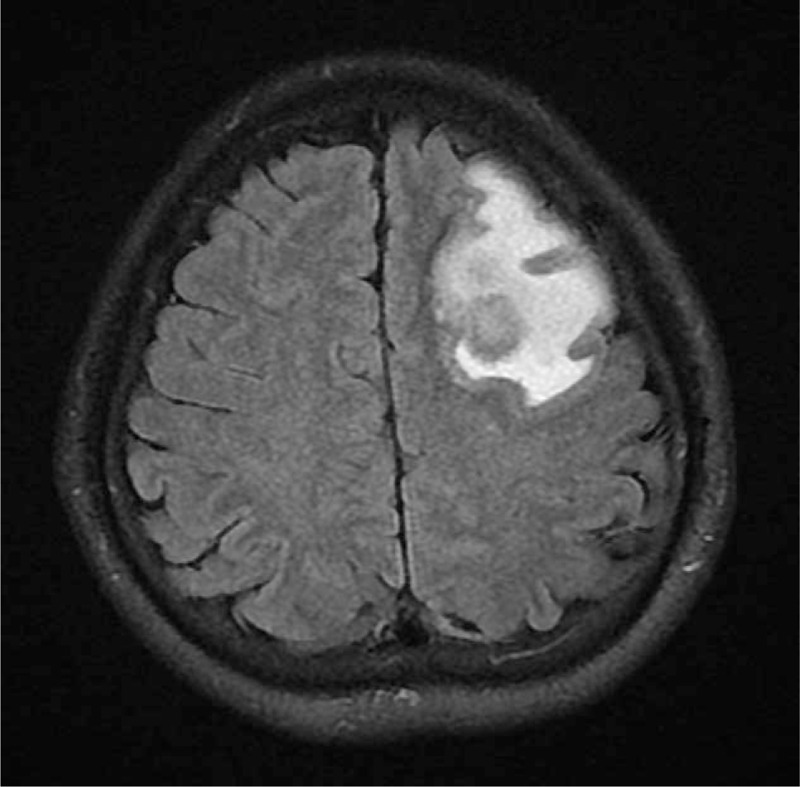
Brain magnetic resonance imaging revealed left frontal lobe abscess.
